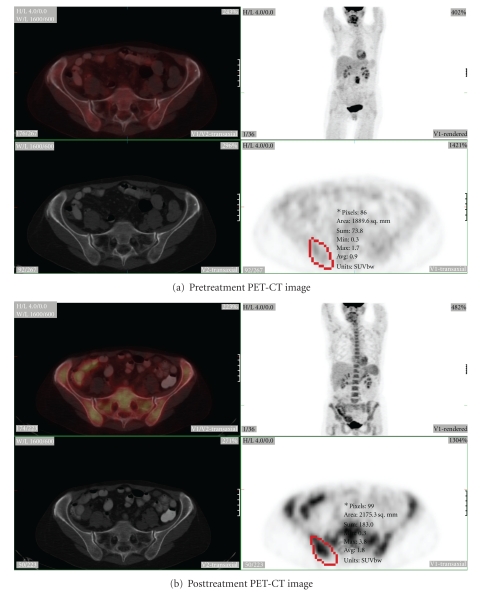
58-year-old female with follicular grade 3 lymphoma (a) without focal evidence of marrow involvement by PET. She had right iliac biopsy. The posttreatment PET-CT (b) again shows diffuse increase in marrow uptake. The SUV increases (from 1.7 to 3.8) on the right iliac bone marrow (red region) with pathologic examination negative for marrow involvement. The change of the SUV over bone marrow is all due to normal hematopoietic response to chemotherapy.
